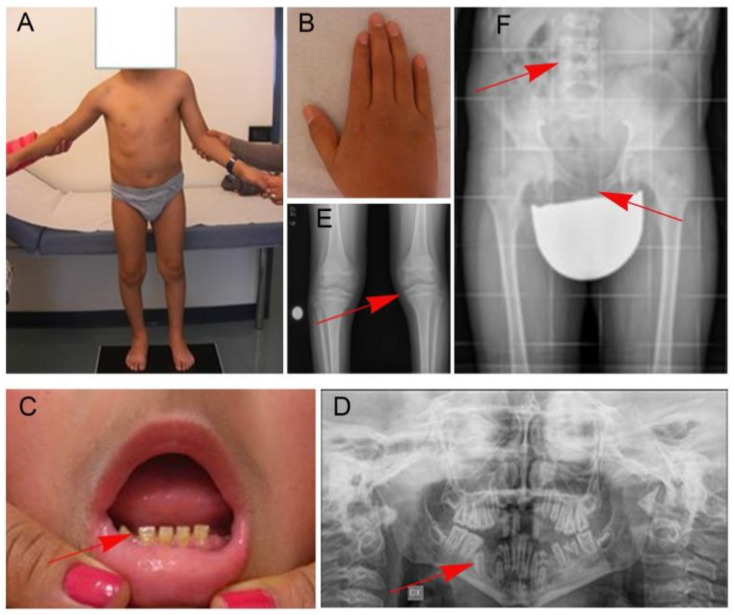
Clinical features of the subject with the homozygous *LTBP3* splice site variant. Proband’s photographs showing short trunk, varus knees, upper limbs diplegia (A) and brachydactyly (B). The subject also showed amelogenesis imperfecta and hypodontia (C). Radiographic examinations of the dental archs, lower limbs, pelvis and trunk demonstrated oligodontia (red arrow in panel (D), slightly widened appearance of the proximal epiphyseal region of the tibiae and of the distal epiphyseal region of the femurs (E), platyspondyly (F).
